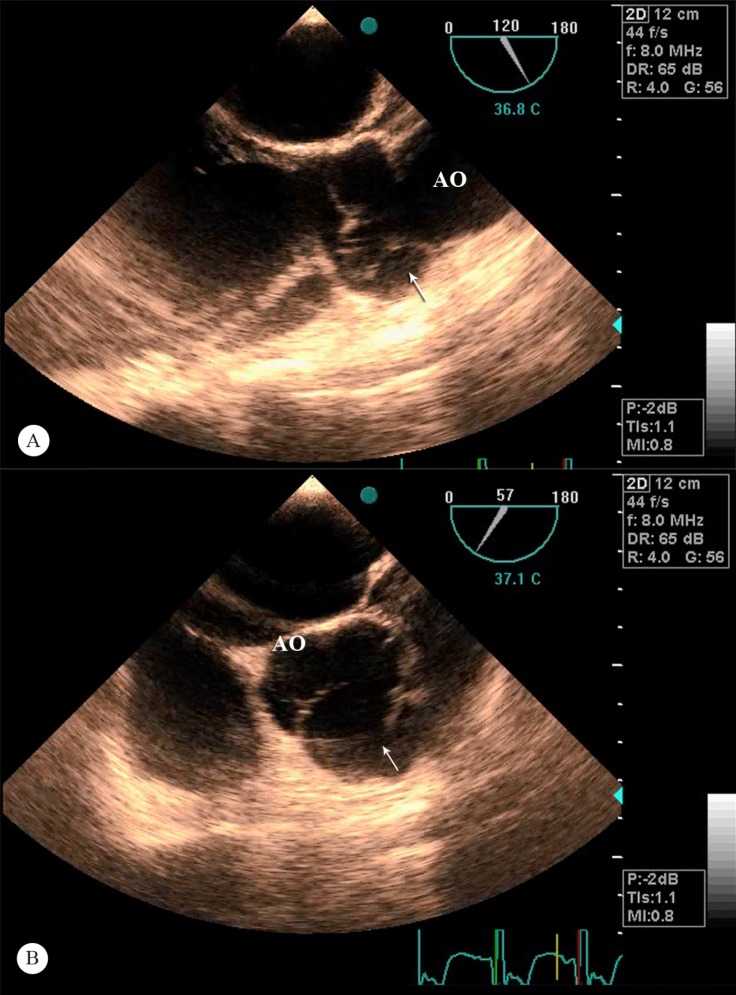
Transesophageal echocardiographic long axis (A) and short-axis (B) views of the aortico-left ventricular tunnel (Arrow) and the relation to the aorta (AO).
